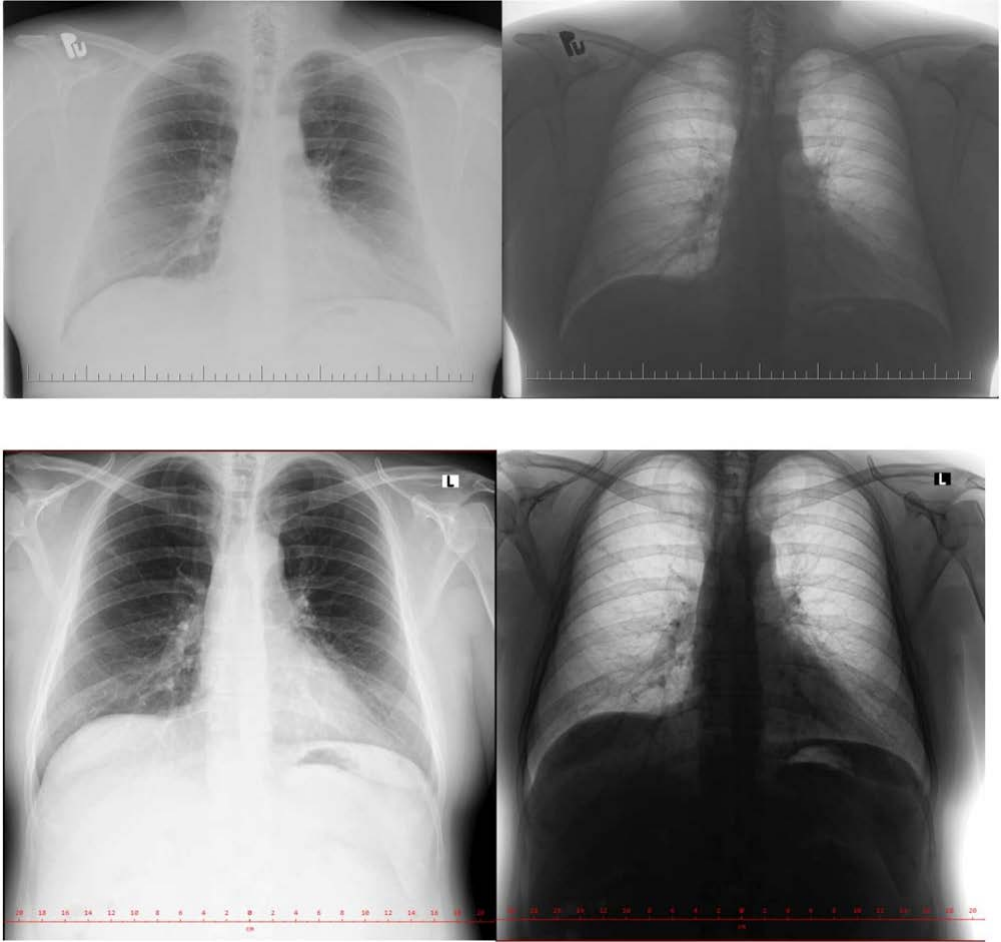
A: Chest X-ray in Posterior-Anterior projection of lungs. May 2019 local hospital’s surgical department. B: Chest X-ray in Posterior-Anterior projection of lungs. March 2021 local hospital’s surgical department.
